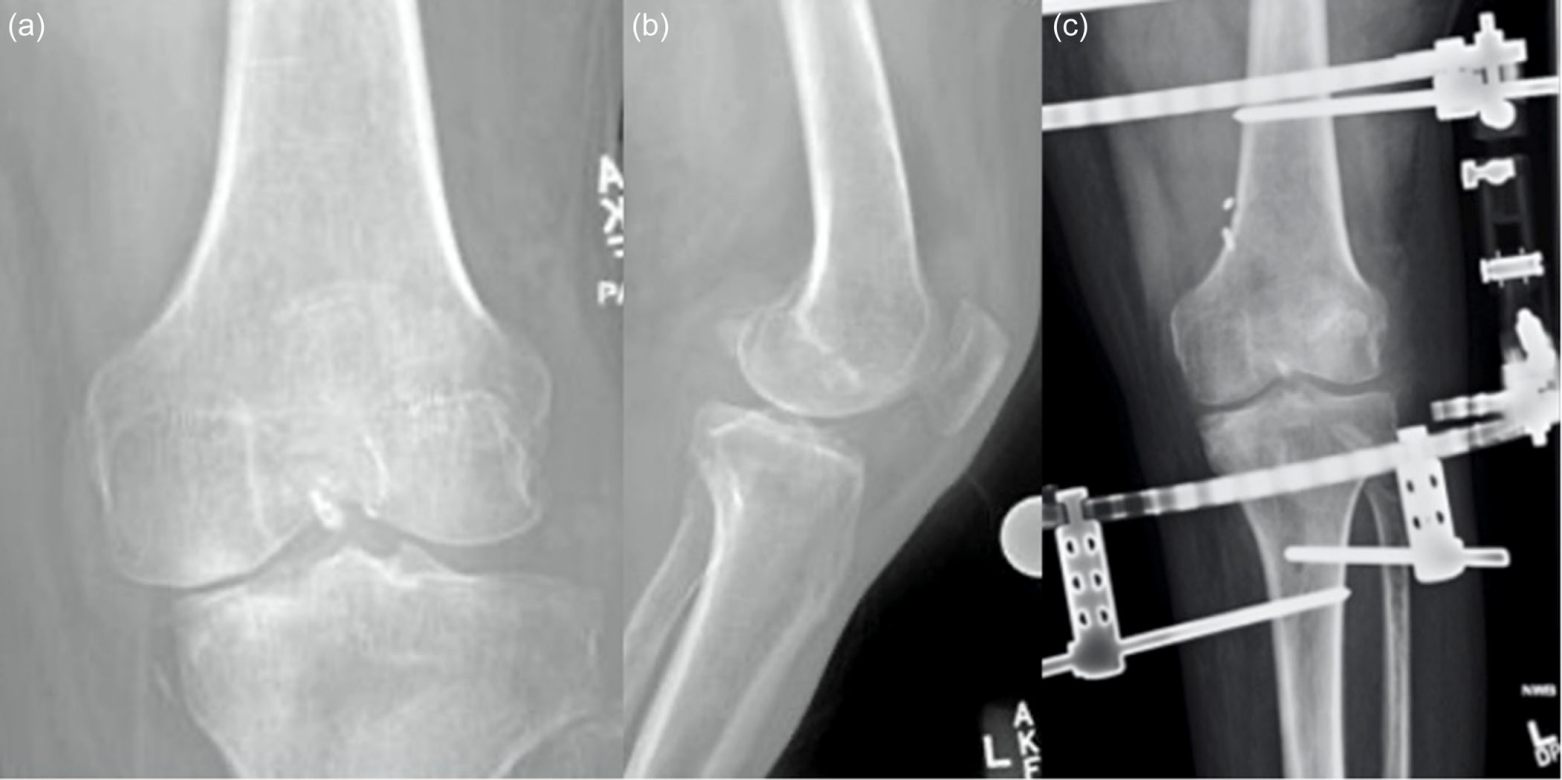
(a) Anteroposterior and (b) lateral radiographs at initial presentation 9 months post‐injury showing substantial lateral tibial subluxation in the coronal plane only. (C) Post‐operative radiograph shows application of hinged external fixator after tibiofemoral reduction and anterior cruciate ligament/posterolateral corner reconstruction.
